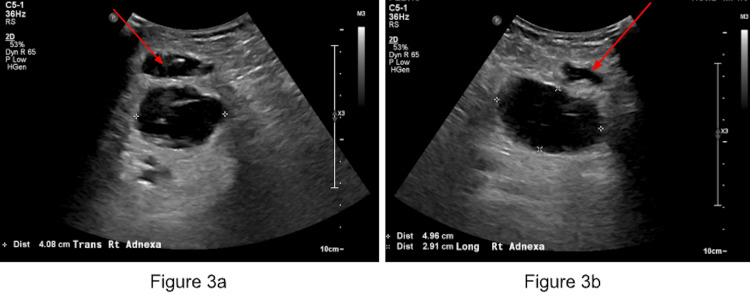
Transvaginal transverse (a) and longitudinal (b) ultrasound images of the right ovary demonstrating a mass (red arrows). This image demonstrates an enlarged right ovary with a complex hypoechoic mass in the right adnexa, measuring 5 cm (red arrows). This is superior to the incision site in the right groin, which could be a hematoma.
